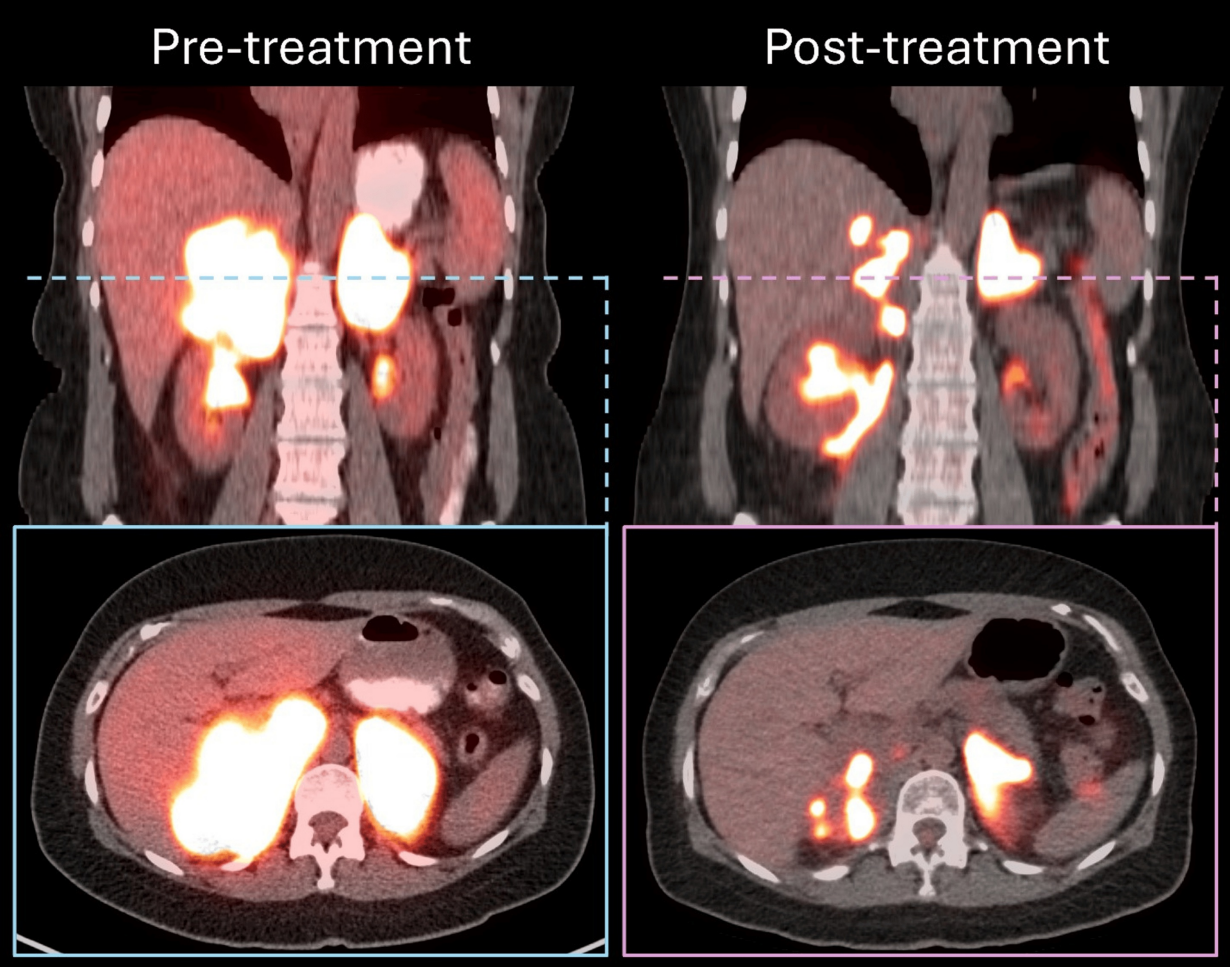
Pre-treatment and post-treatment PET/CT scans, showing the response to online adaptive radiotherapy.
